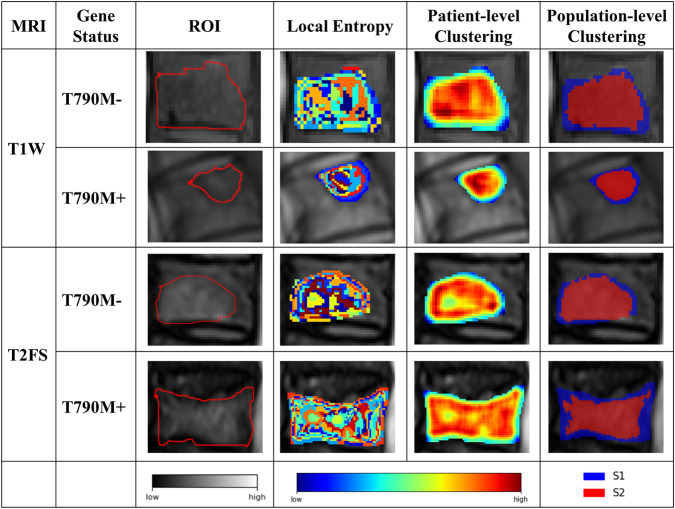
Results of the intratumor partition in the T1W and T2FS MRI. The ROI column represents the MRI images with manually segmented spinal metastases. The local entropy column represents entropy maps of the metastases.
